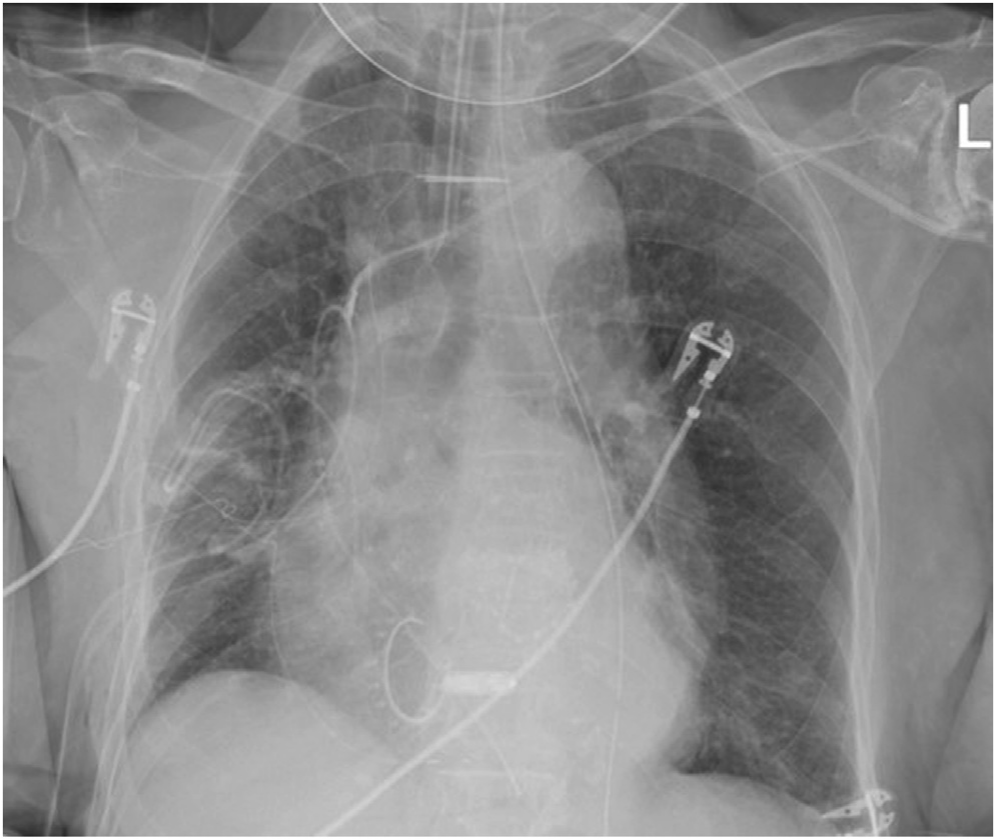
Patient 1 chest radiography on postoperative day 1, which demonstrates a well-positioned leadless pacemaker and tricuspid and mitral rings.
